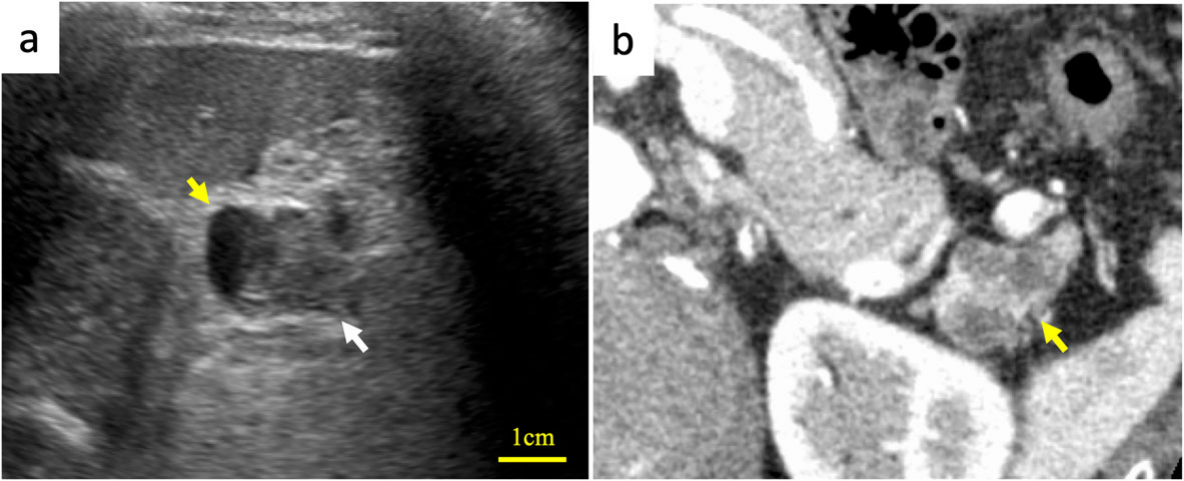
a Abdominal ultrasound showing a cystic lesion in the pancreatic tail (yellow arrow) and a slightly hyperechoic component (white arrow) in the cystic lesion. b Contrast-enhanced abdominal computed tomography shows a cystic mass with a diameter of 24 mm (yellow arrow)
